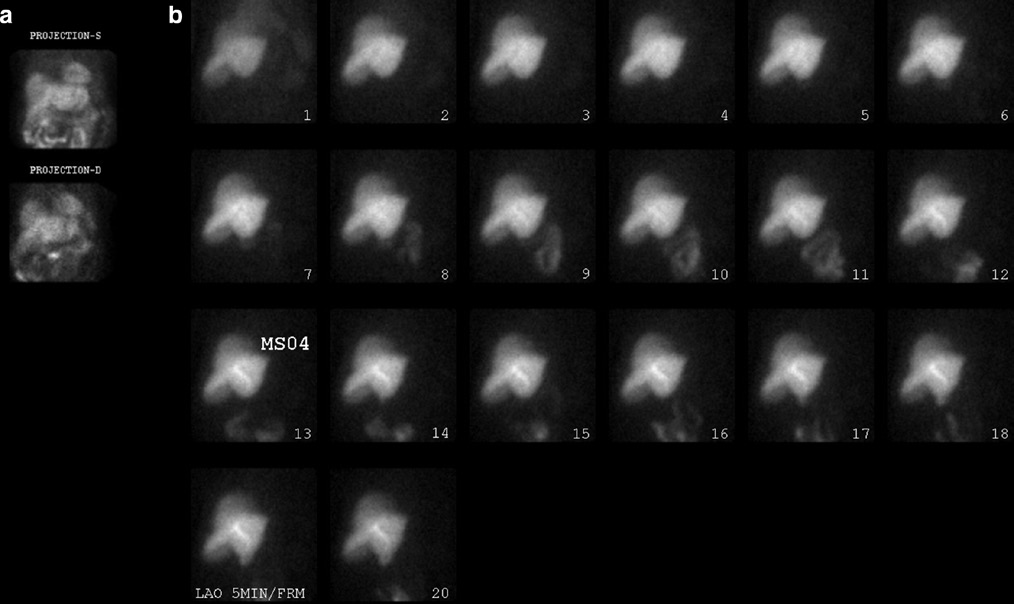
GB not visualized on either the stress/rest MIBI (a) or HBS post MS04 data (b). GB, gall bladder; HBS, hepatobiliary scintigraphy; MIBI, methoxy isobutyl isonitrile.
